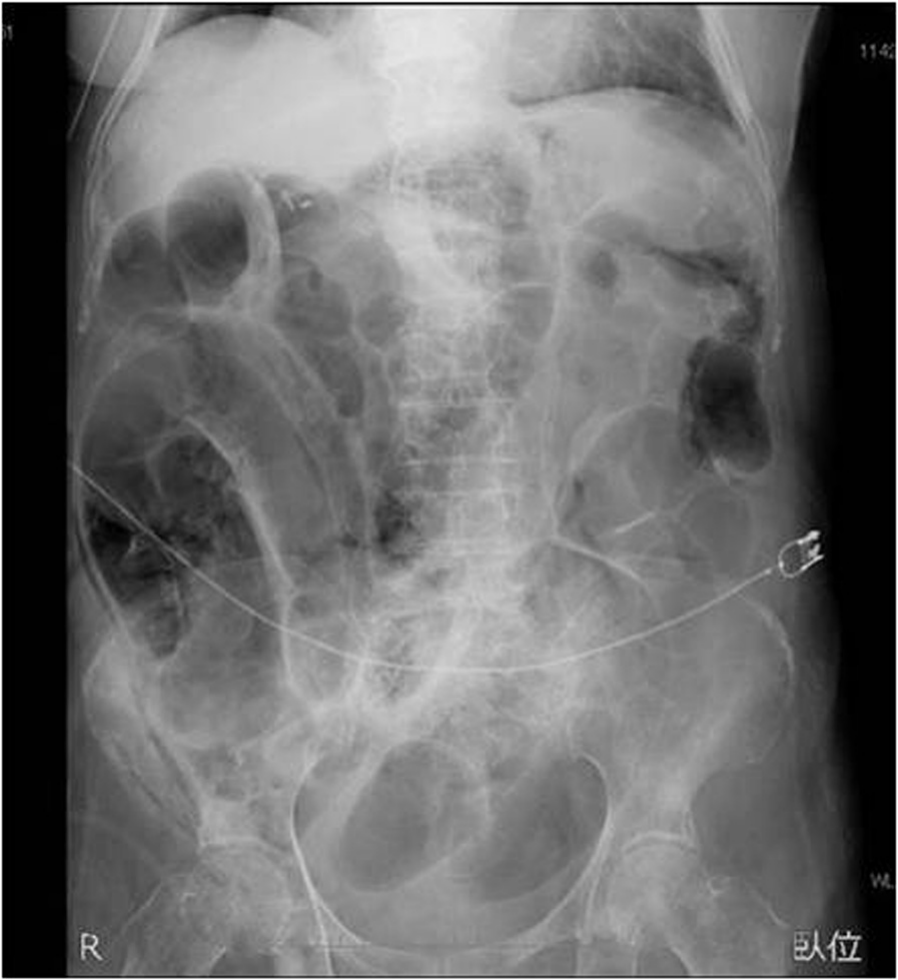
Abdominal X-ray performed after treatment revealed decreased intraluminal gas
